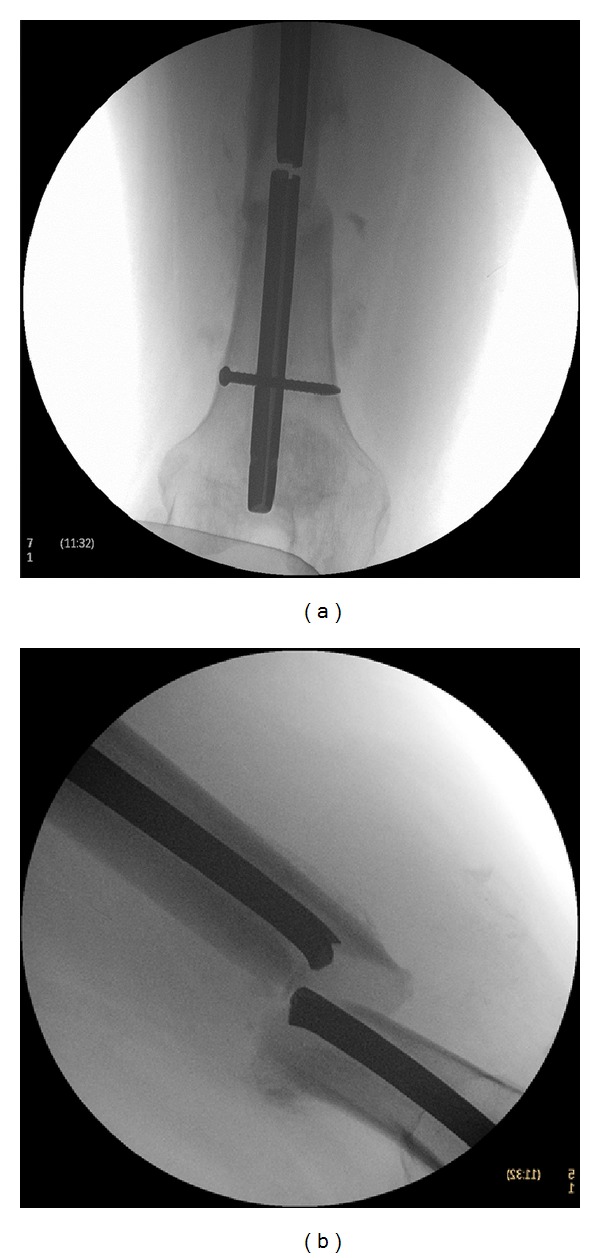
The radiographs show displaced fracture at distal 1/3 of the right femur with a retained broken AO femoral nail in (a) AP and (b) lateral view.
